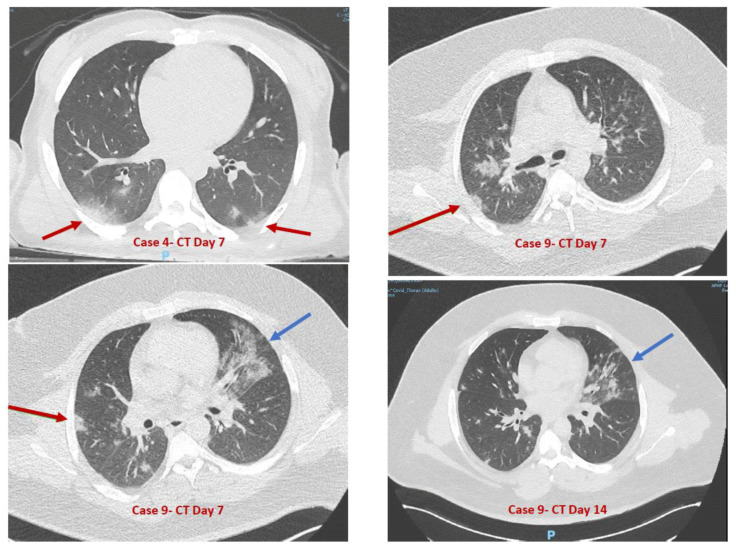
Chest CT (computerized tomography) scan of cases 4 and 9. The red arrow shows images of ground glass opacities; the blue arrow shows the area of secondary infection.
